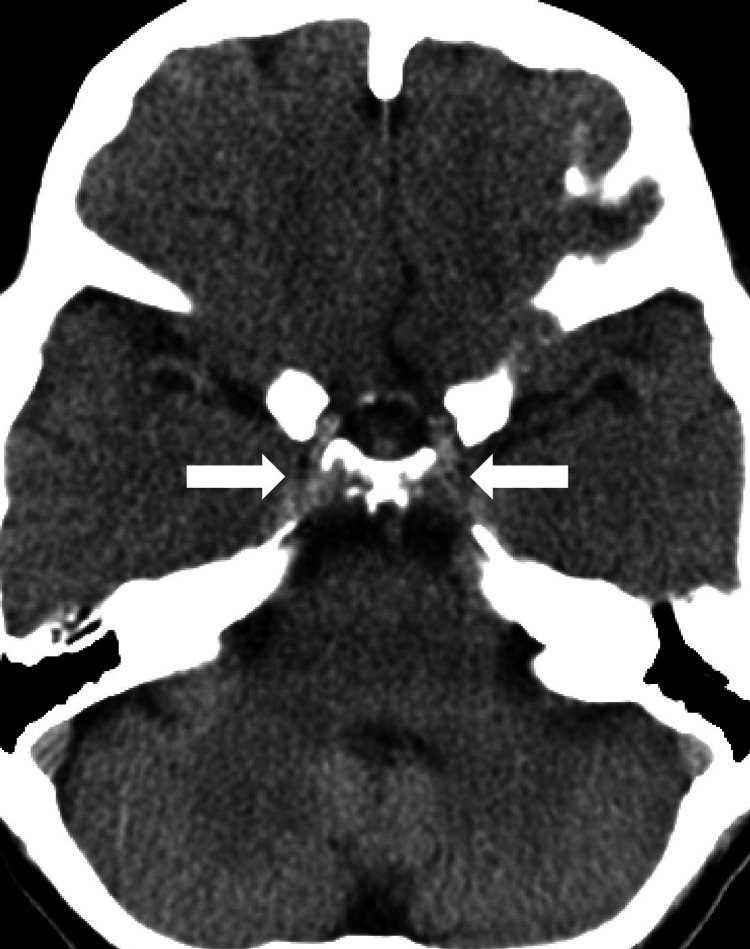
Computed tomography of the head showing fullness in the bilateral cavernous sinus.
